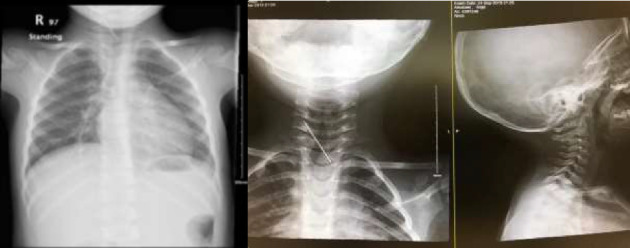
Chest X-ray showing a 4 cm, hyperdense foreign body projecting over the posterior lower neck.
